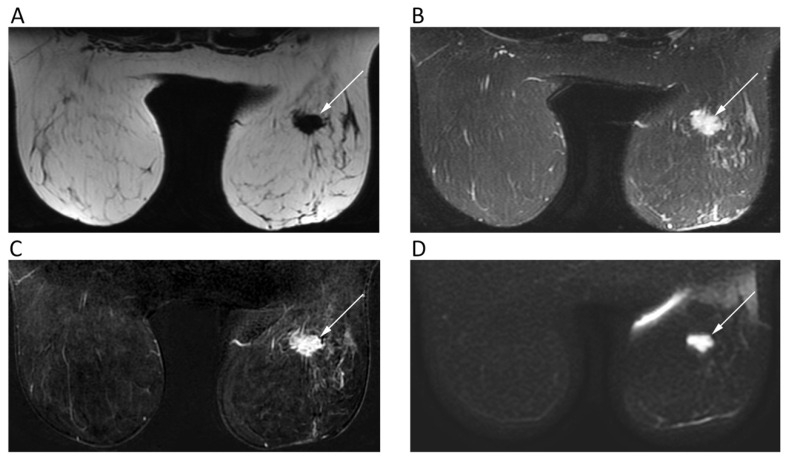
Breast MRI of a 42-year-old female patient with right breast carcinoma. Axial IDEAL fat only (A) and IDEAL water only (B) images show a right breast carcinoma (arrows) with strong enhancement on post-contrast T1w image (C) and high signal intensity on *b* = 900 DWI image (D).
